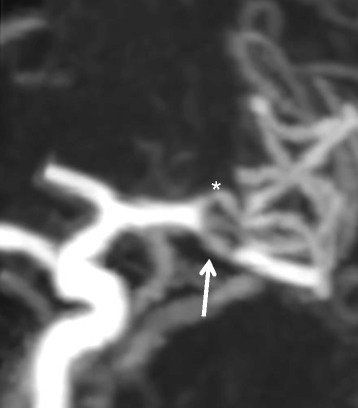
Noninvasive imaging. Three-dimensional 360-slice CTA of the head of a 50-year-old man presenting with abrupt-onset 2-day history of headache and right hemiparesis demonstrating left M1 dissection (arrow) distal to the anterior temporal branch (asterisk). He was discharged on antiplatelet therapy with a modified Rankin Scale score of 0.
